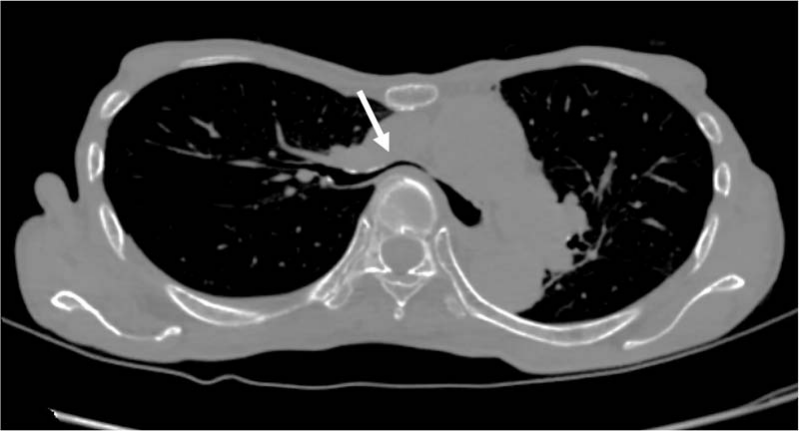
Chest CT findings of severe PE with stenosis of the right main bronchus. The right main bronchus was severely narrowed with a HI (HI) of 8.9 (arrow).
